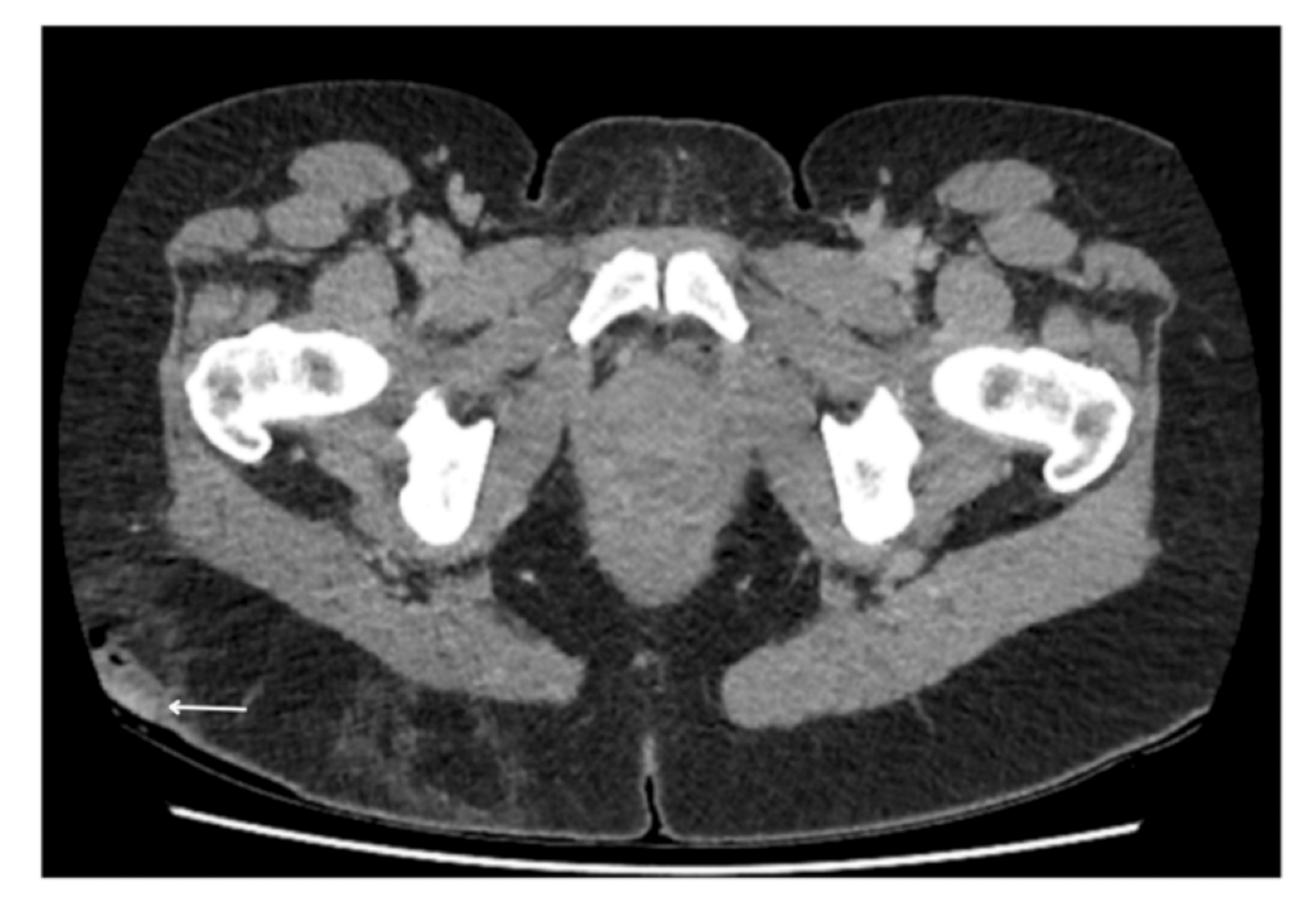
Axial post-contrast venous phase CT scan image of the pelvis. Small area of subcutaneous density containing small gas pocket in keeping with fat necrosis (arrow)
